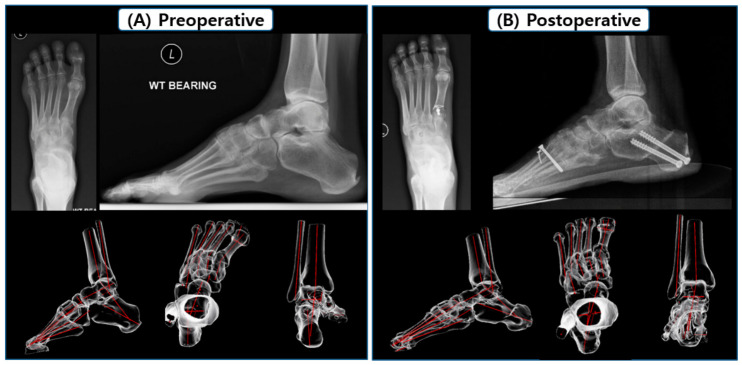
Preoperative and postoperative imaging findings of the left foot in Charcot–Marie–Tooth patient who underwent joint-sparing surgery. (A) Preoperative and (B) postoperative standing foot radiographs, WBCT-based reconstructed 3D models demonstrating the segmental bone axes (red lines) automatically generated by the software for multiplane alignment analysis.
